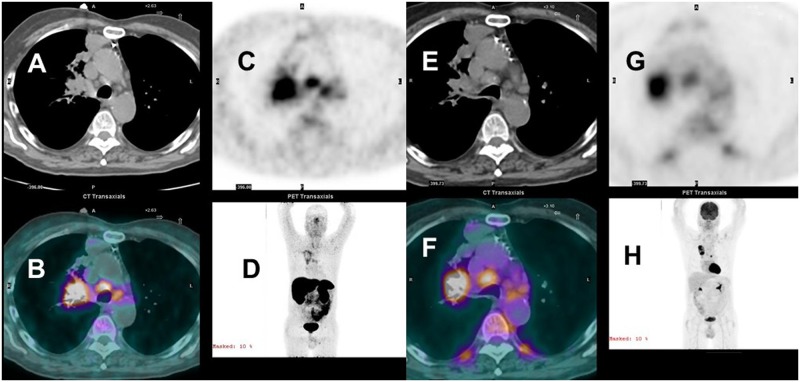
^68^Ga-DOTATATE (A-D) and ^18^FDG PET/CT (E-H) concordant staging stage IIIB squamous cell carcinoma. Concordant uptake in the right upper lobe tumor, right hilum and mediastinal adenopathy. Axial CT images (A&E) at mid chest with emission (C&G) and fused images (B&F). Anterior 3D maximum intensity images, D&H.
